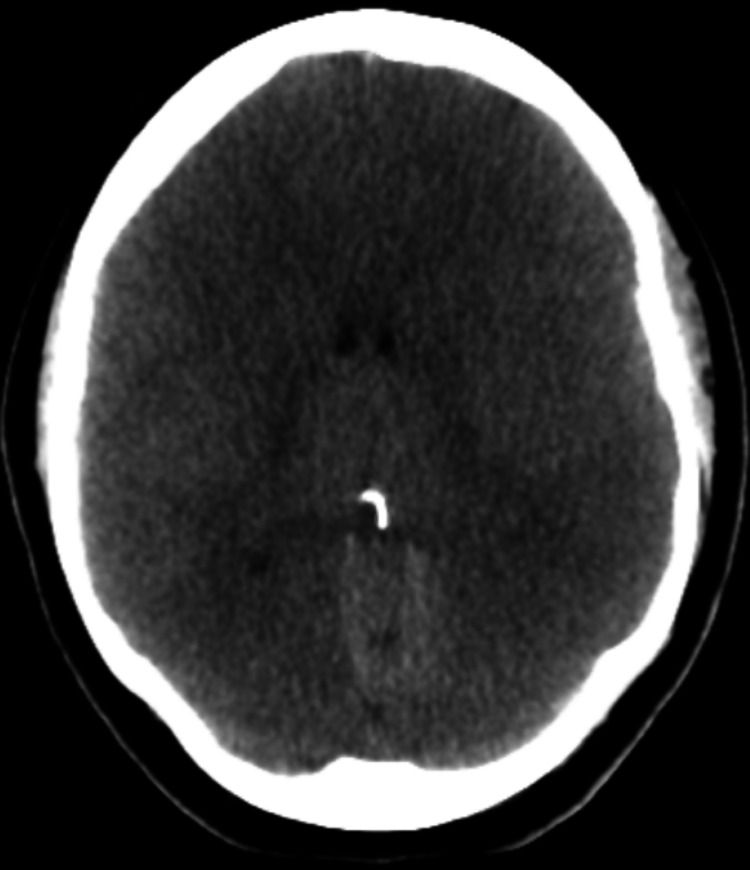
CT of the brain on hospitalization day three showing severe diffuse cerebral edema CT: computed tomography
